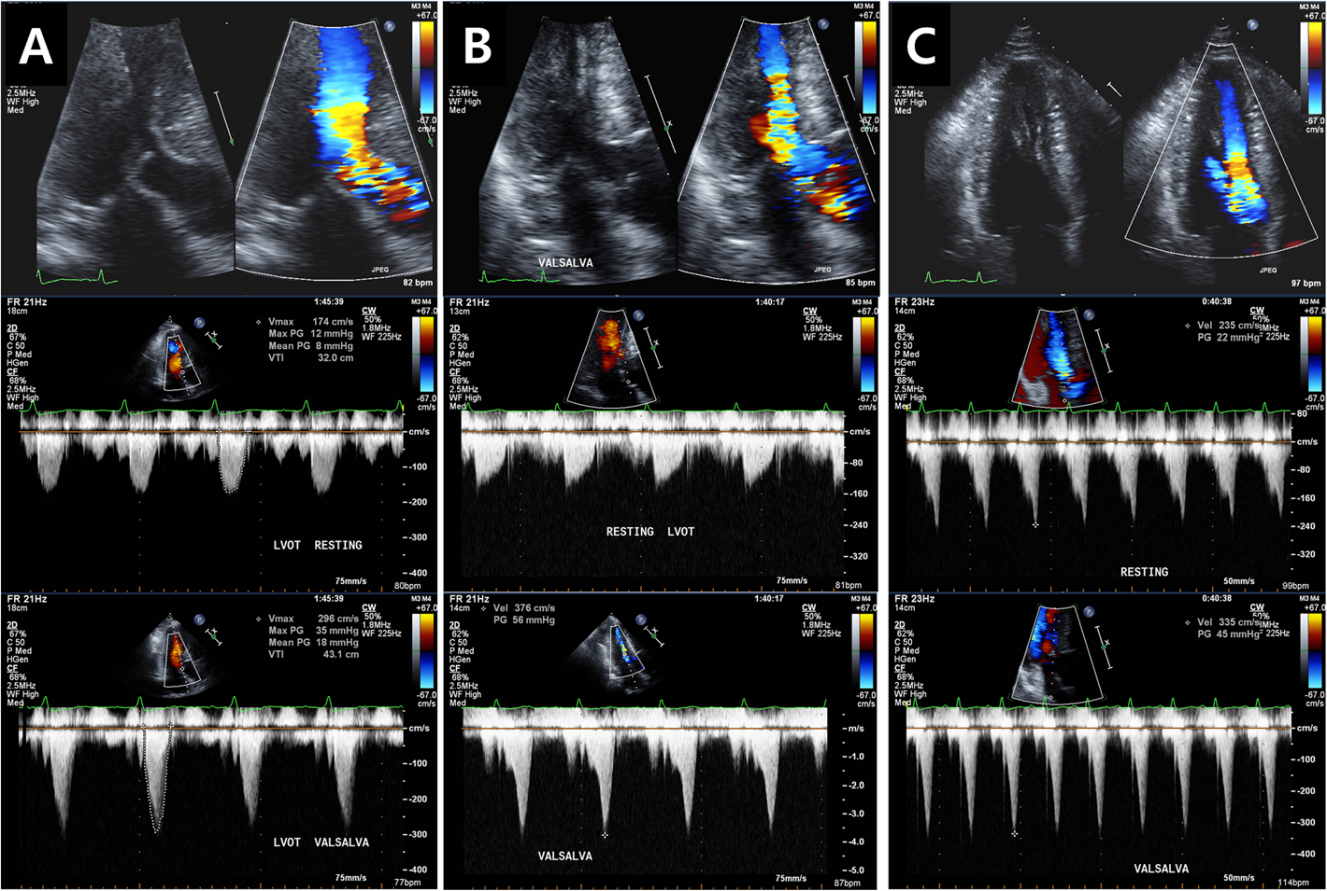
Representative cases of DLVOTO with Doppler patterns at rest and during Valsalva maneuver. A case with leaflet systolic anterior motion (SAM) (A), a case with chordal SAM (B), and a case with prominent papillary muscles kissing to septum (C).
